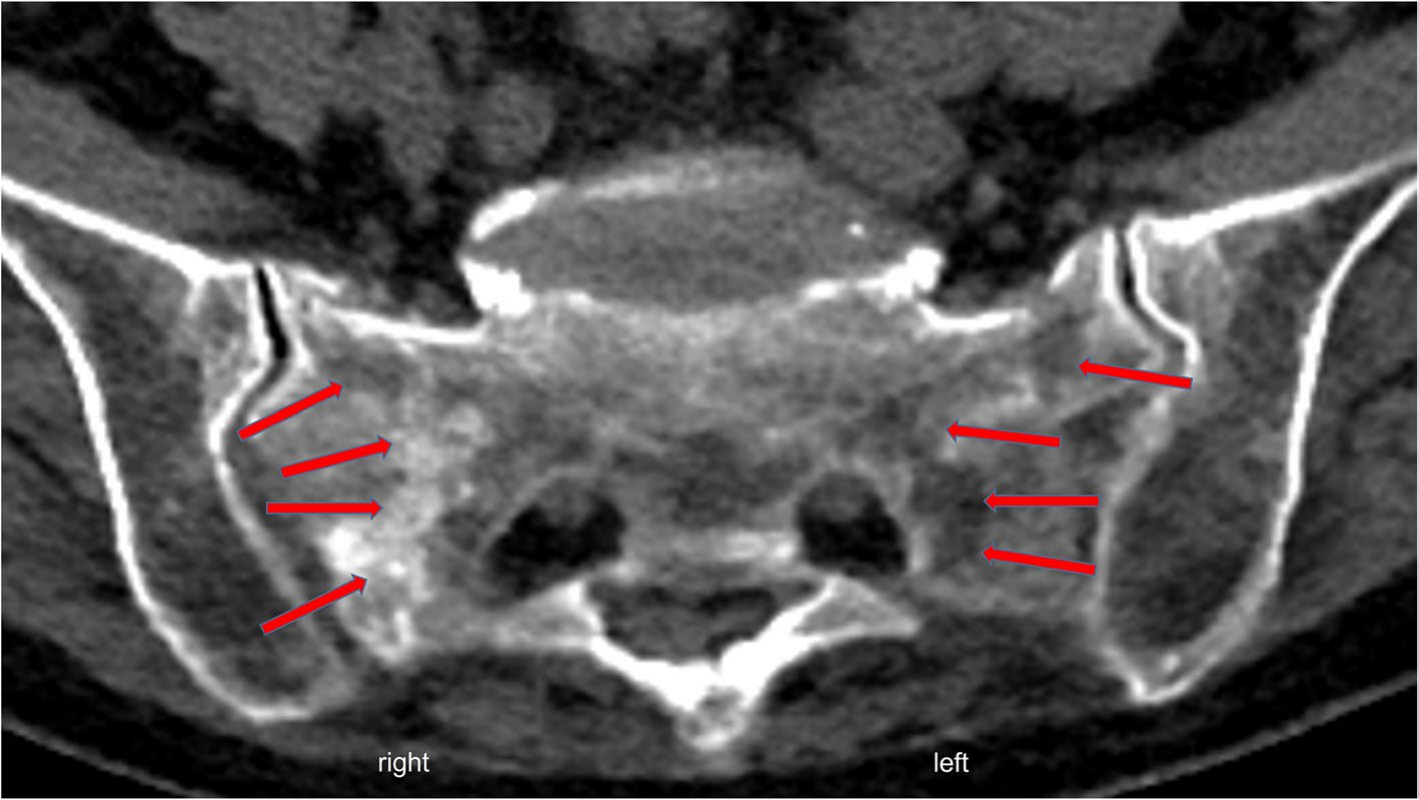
Axial CT slice shows a bilateral FFS of different ages with marked sclerosis in the fracture zone on the right and without remodelling reactions in the fracture zone on the left
